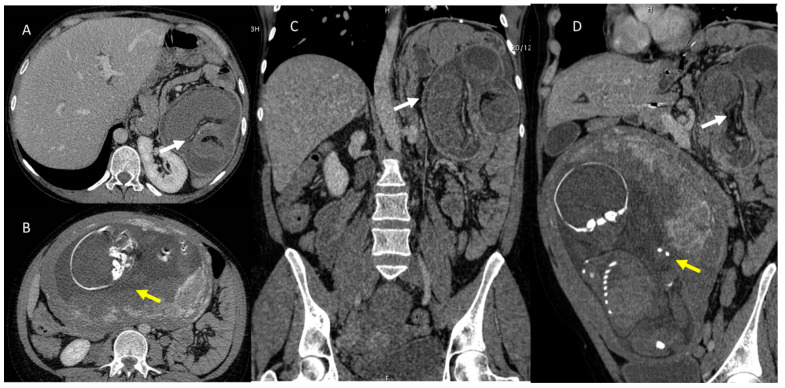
Pregnant woman (yellow arrows) with emergency room admission for abdominal pain, vomiting, and heart rhythm disturbances. US assessment reveals no specific findings. CT study in axial plane (A,B) and in coronal MPR plane (C,D) shows volvulus (white arrows) of the small bowel in the left epigastrium.
